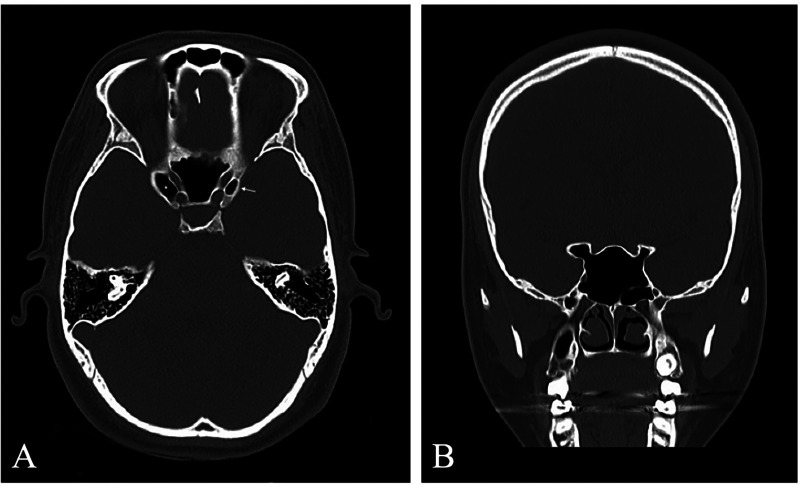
Type 3b anterior clinoid process (ACP) pneumatization (A) axial CT image demonstrating right ACP pneumatization through the planum sphenoidale with >50% ACP involvement (type 3b, asterisk). (B) Coronal CT image showing pneumatization extending from the sphenoid sinus through the planum sphenoidale into the ACP (Type 3b, asterisk).
